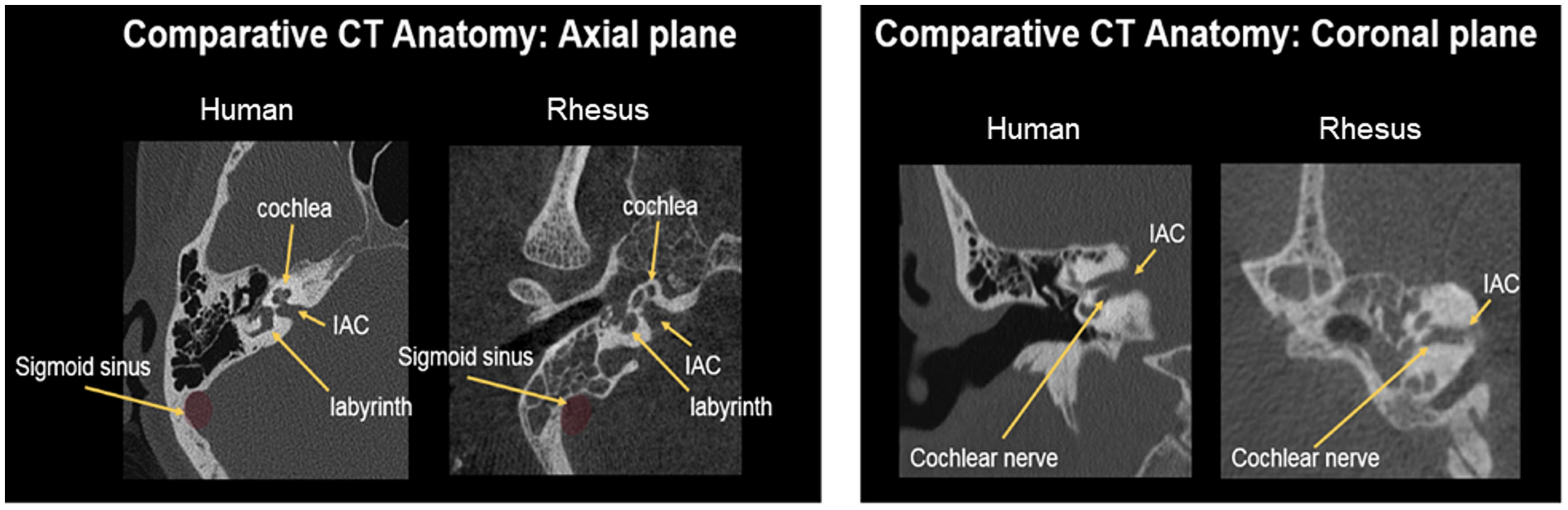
Computed tomography scans of the NHP and human inner ear anatomy. Key structures such as the internal auditory canal (IAC), sigmoid sinus, and cochlea reside in similar relative locations, facilitating translation of the translabyrinthine surgical access procedure. The nerve bundle within the IAC can be readily accessed via drilling through the surrounding labyrinth.
